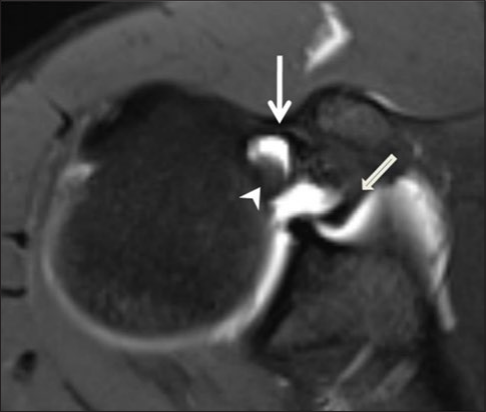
Pulley system. Axial fat-saturated T1-weighted MR arthrographic section at the level of the bicipital groove shows the biceps pulley (large arrow), formed by the fusion of the coracohumeral ligament, the superior glenohumeral ligament (thin arrow) and the transverse humeral ligament. The long head of the biceps tendon is pointed out by an arrowhead.
